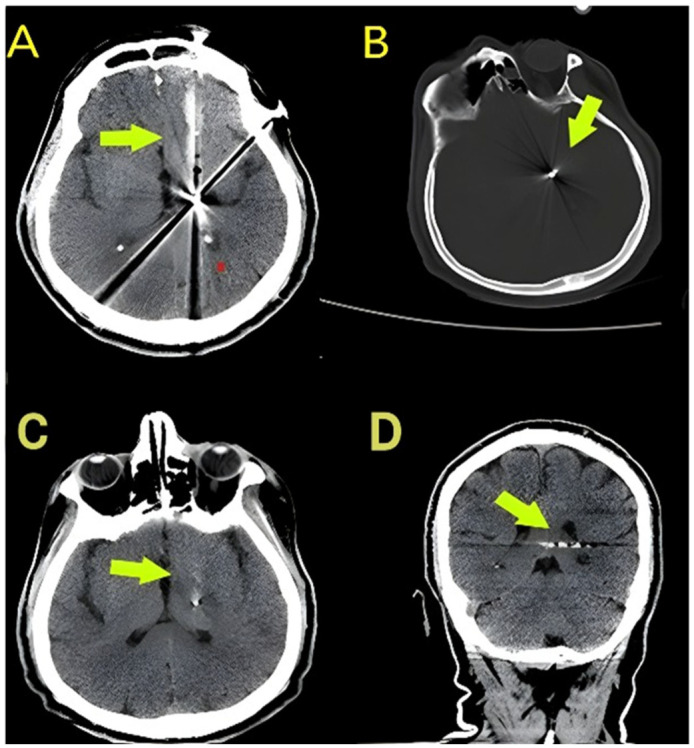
Representative admission CT images demonstrating an anatomical burden score of 1. (A) Axial non-contrast CT showing a projectile tract confined to the frontal lobe with associated parenchymal haemorrhage (arrow). (B) Axial bone-window CT demonstrating the retained metallic fragment without cross-midline extension (arrow). (C) Axial CT image showing deep structure involvement along the projectile tract (arrow). (D) Coronal reconstruction confirming absence of intraventricular haemorrhage and no cross-midline trajectory (arrow). The anatomical burden score was 1, reflecting deep structure involvement without multilobar extension, cross-midline trajectory, or intraventricular haemorrhage.
